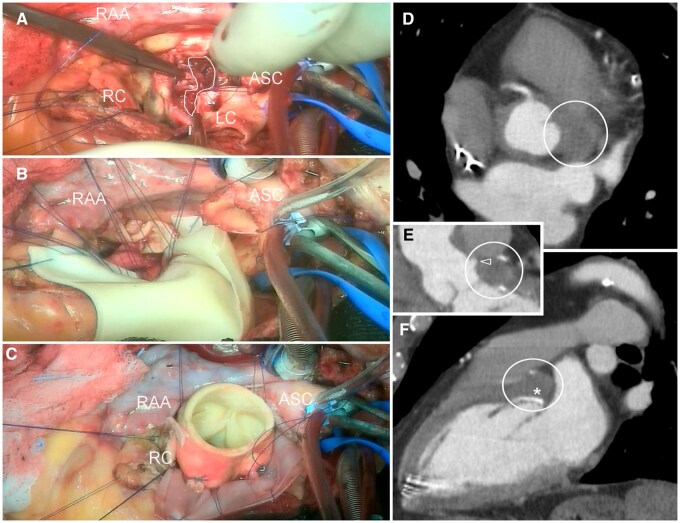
(A-C) Intraoperative View. (D-F) Angio-CT after 1 Year (Asterisk: xeno-Pericardial Patch in the LVOT; Triangular Arrow: Sub-Occlusion of the Left Main Stem).
